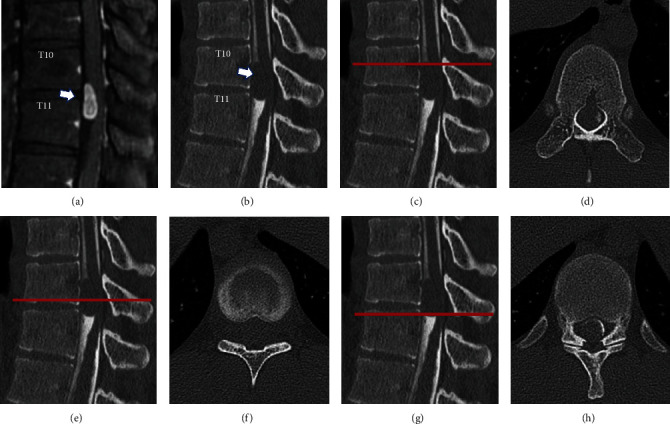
Magnetic resonance imaging before myelography (a) and computed tomography after the myelography (b) shows the intradural mass rostrally migrated with approximately 10 mm discrepancy in the exact location compared to magnetic resonance imaging findings. Arrows indicate the location of the lesion. Each level of axial image on computed tomography shows the proximal (c, d), middle (e, f), and distal (g, h) levels of the tumor.
